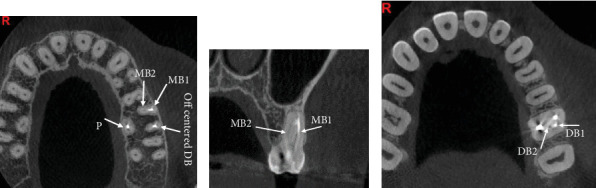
(a, b) Axial view of the CBCT revealing two distinct mesiobuccal canals (MB1 and MB2) along with an off-centered obturated distobuccal canal. (c) Coronal views of Tooth #15 highlighting the missed canals.
